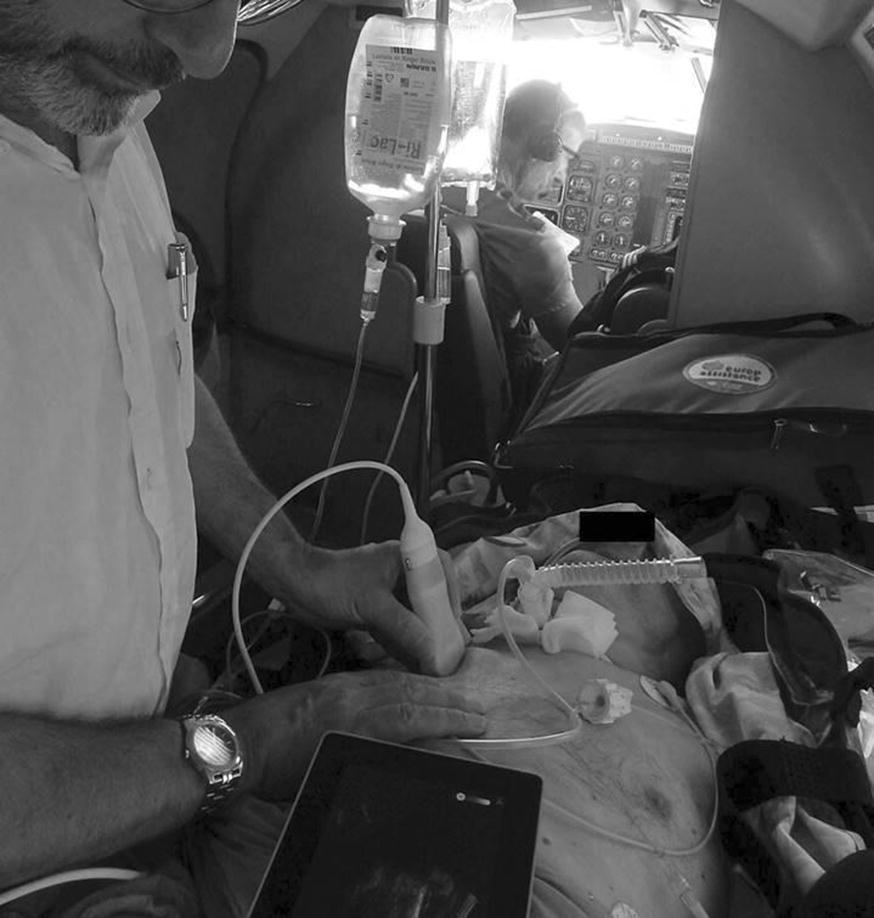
One single machine, well operated, can replace more than just radiography, even CT in strategic areas (lungs mainly). The small size, the light weight are mandatory criteria for life-saving use in this airplane, but the same spirit can be found at ground level, in ICUs or many other settings
